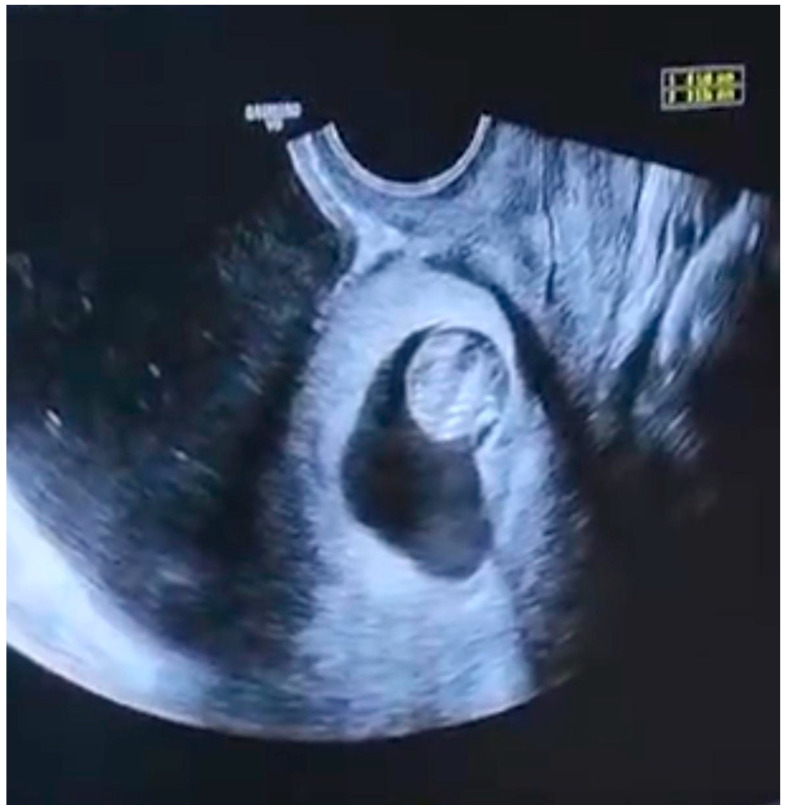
Preoperative transvaginal ultrasound image showing a cesarean scar pregnancy (CSP) sac implanted within the lower uterine segment (Type II CSP according to Timor-Tritsch classification). The scale bar indicates 10 mm.
